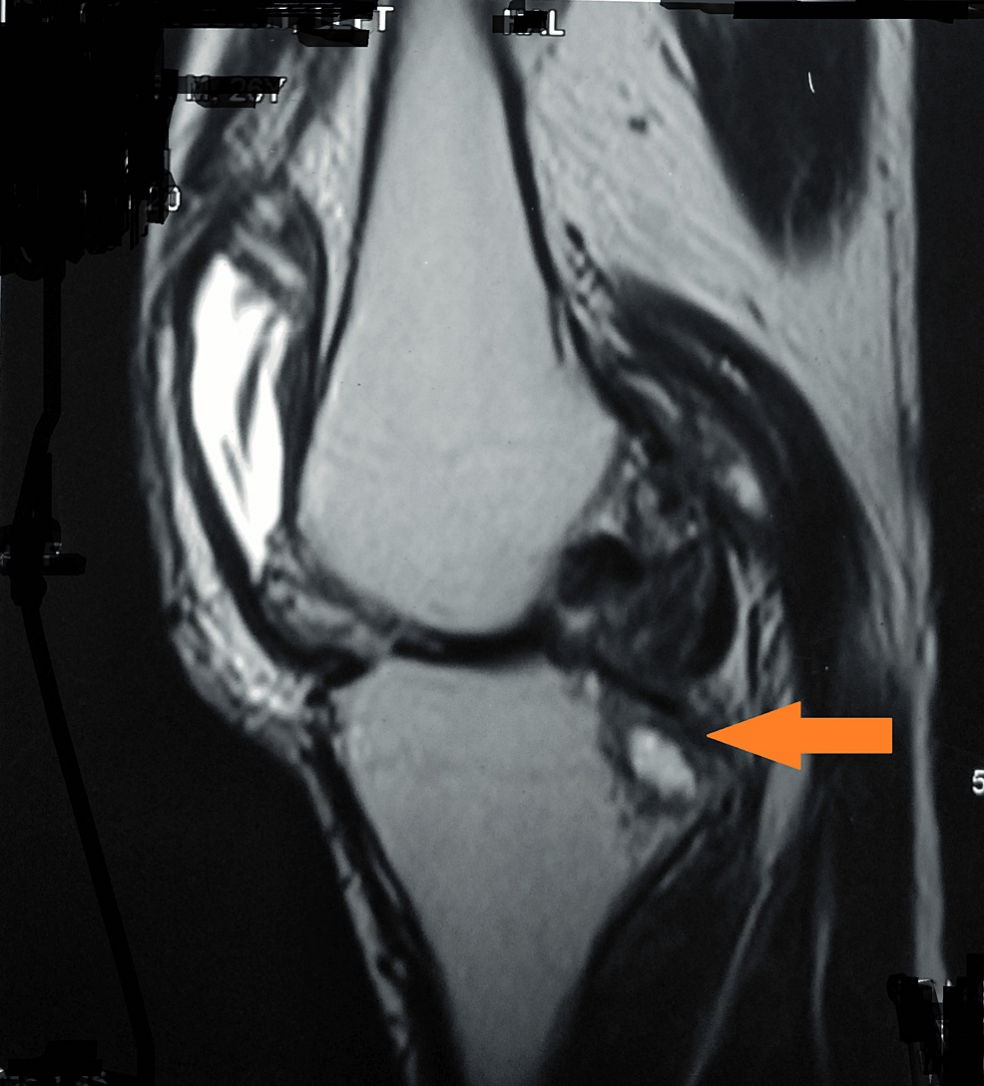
MRI of the left knee joint showing involvement of the knee joint MRI: magnetic resonance imaging
